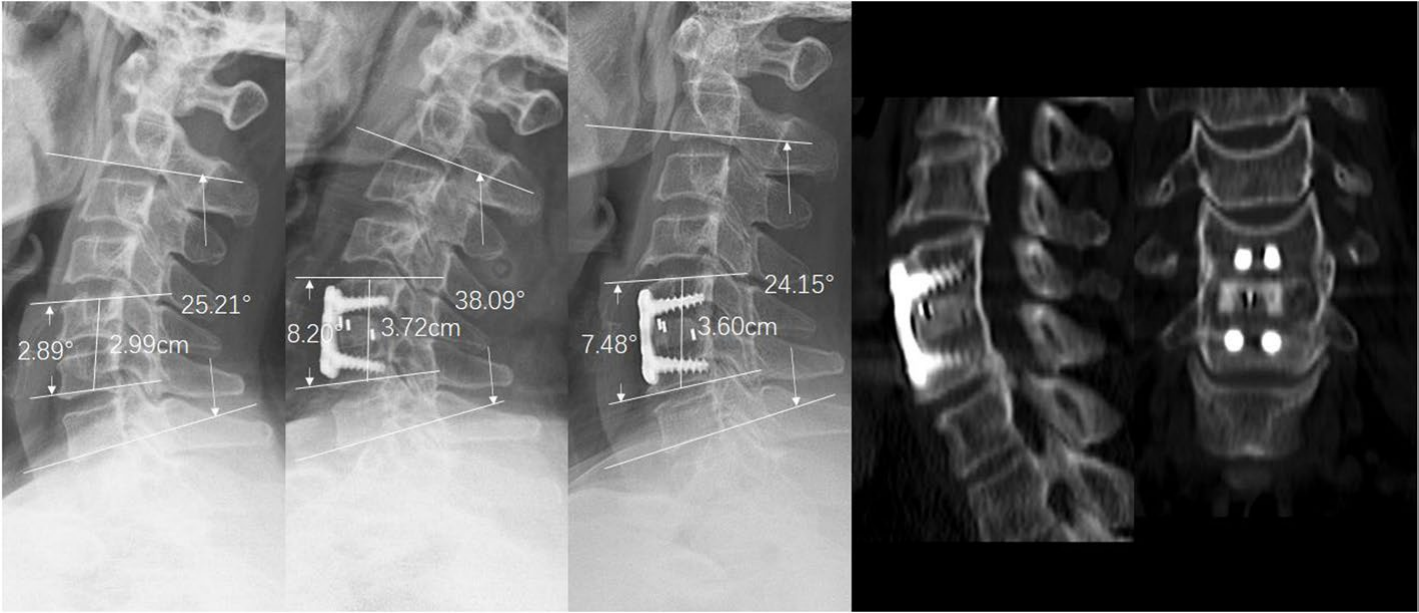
A 49-year-old female patient underwent ACDF with the PEEK cage due to C5/6 disc herniation. a Preoperative lateral radiograph of the cervical spine and narrowing of the disc space between C5/6. b Postoperative radiograph indicated that the SA and IH improved. c The 8-year follow-up radiograph showed stable SA without subsidence. d, e Three-dimensional CT at the 8-year follow-up showed grade 5 fusion. ACDF, anterior cervical decompression and fusion; SA, segmental lordosis; IH, interbody height; CT, computed tomography; PEEK, polyetheretherketone
